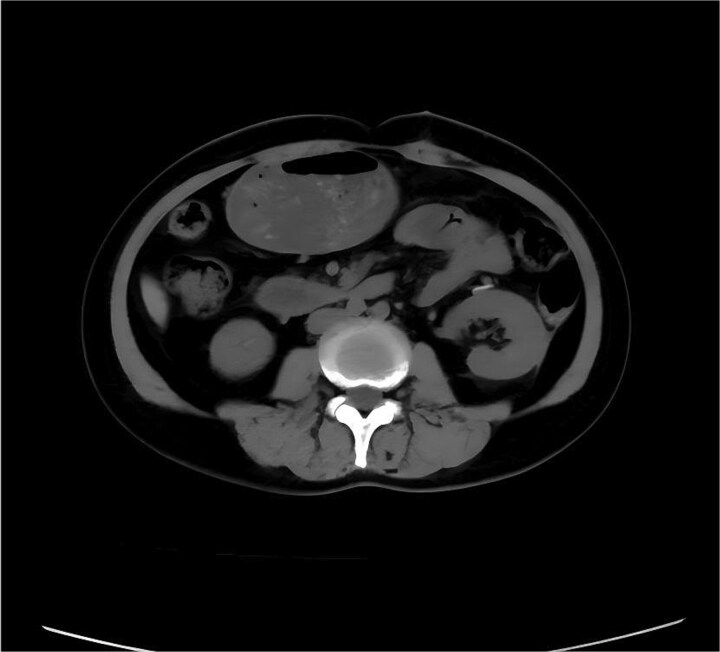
The CT scan showed no recurrence after 12 months following surgery.
